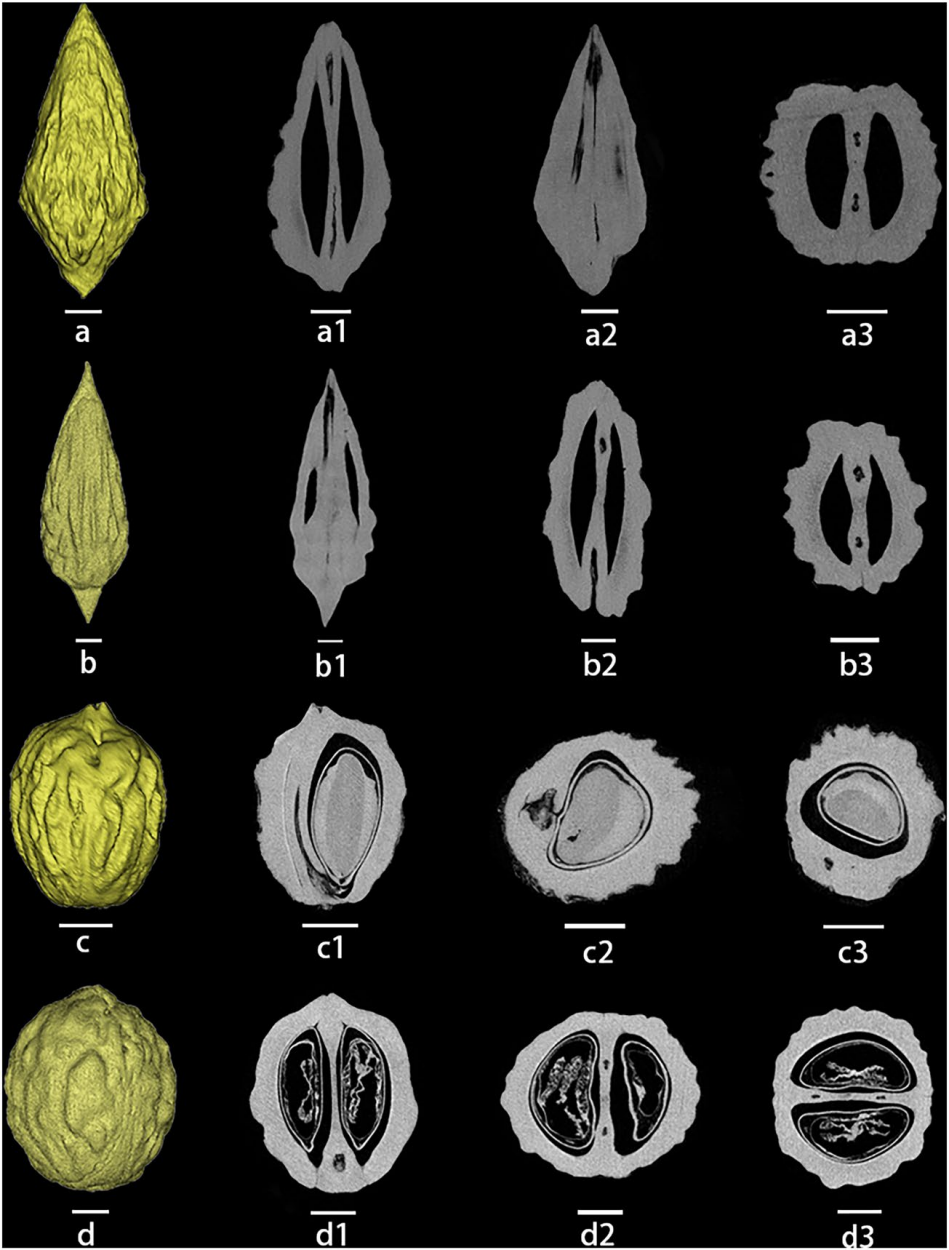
Three-dimensional structures reconstructed using CT and the internal structure of modern jujube (a, b) and sour jujube (c, d) in different views. (a) Zanhuangdazao; (b) Banzao; (c, d) Sour jujube. Scare bar = 2 mm.
